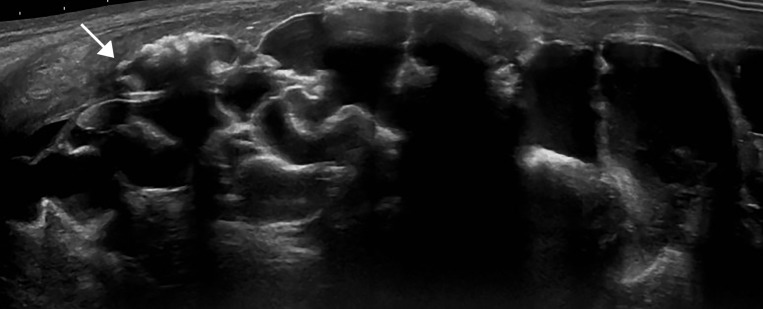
Right hip ultrasound Grayscale ultrasound image demonstrating large, echogenic, lobulated calcifications (arrow) in the lateral right hip.
